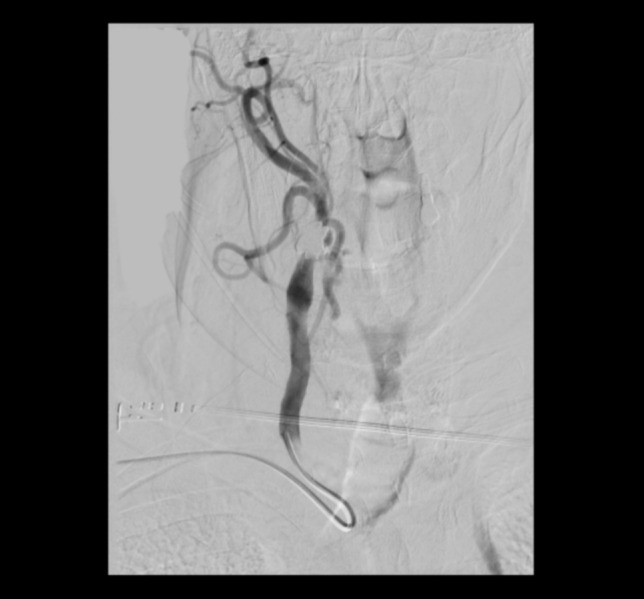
The CT angiography confirmed a significant occlusion in the right internal carotid artery and progressive sharpening of the lumen with an intimal flap due to a carotid dissection
